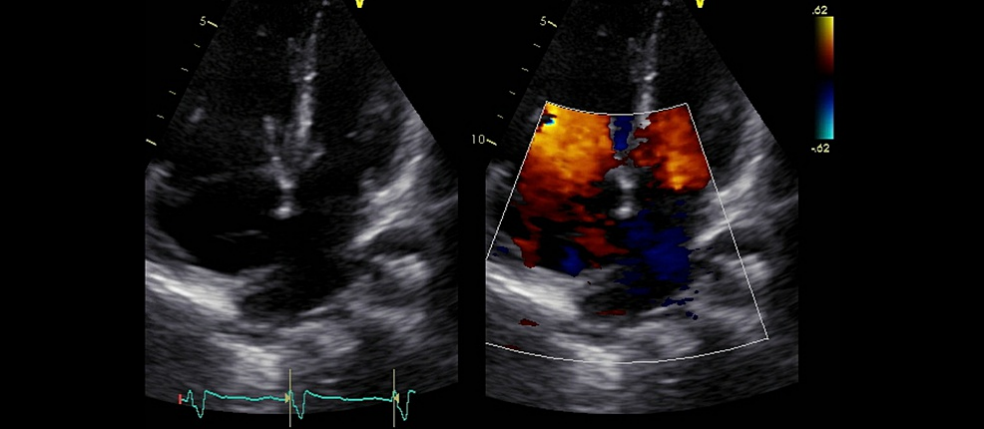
Transthoracic echocardiogram demonstrates a large secundum atrial septal defect with bidirectional interatrial shunt, predominately from left to right. There is moderate right atrial enlargement.
